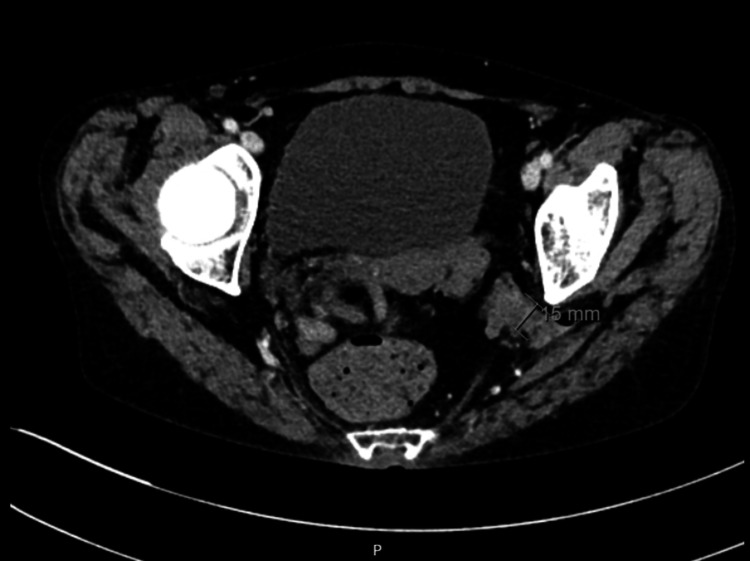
Axial CT image showing a left-sided sciatic hernia measuring approximately 15 mm, protruding through the greater sciatic foramen.
